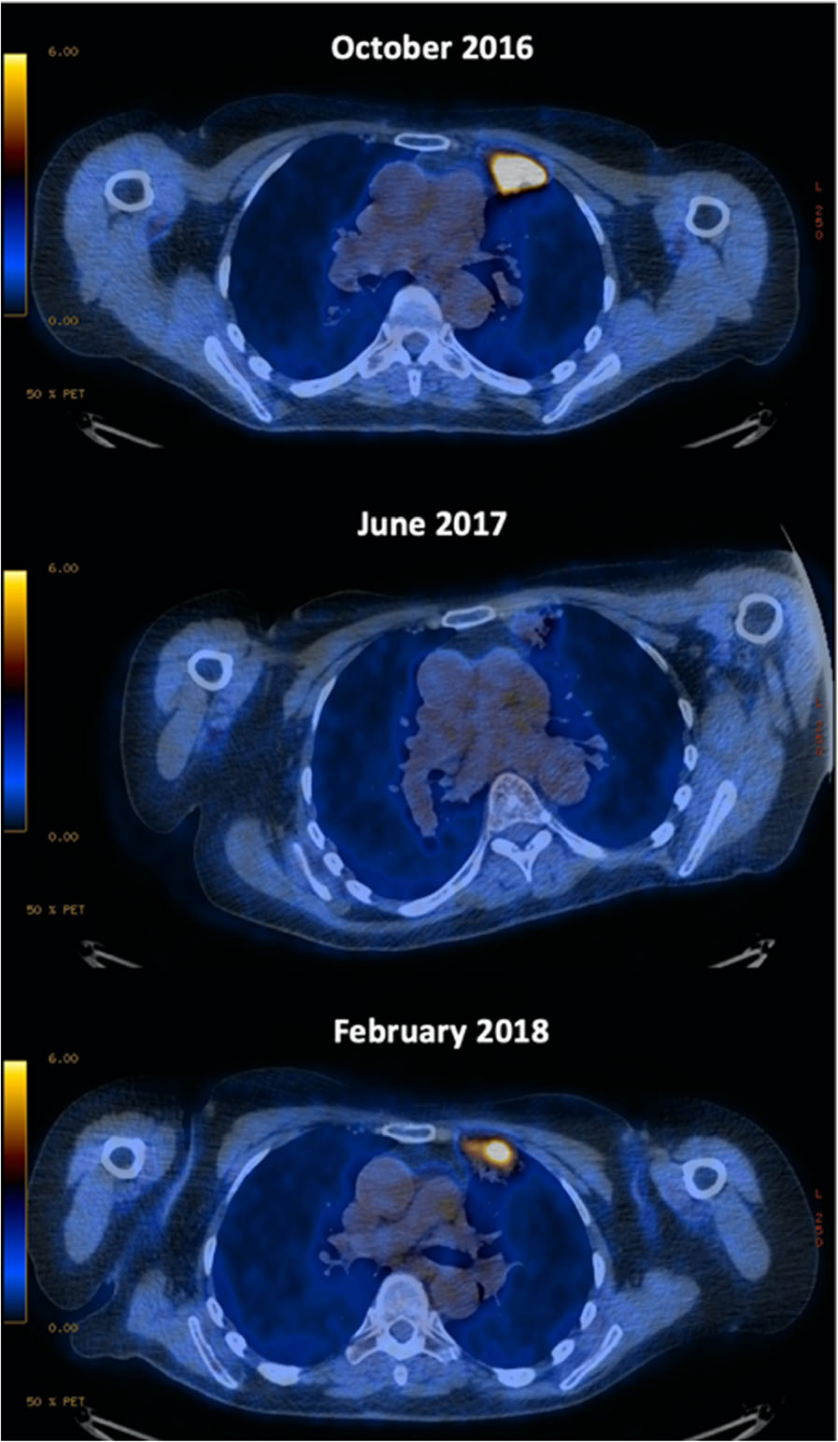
Serial PET-CT appearance of the left upper lobe lesion
